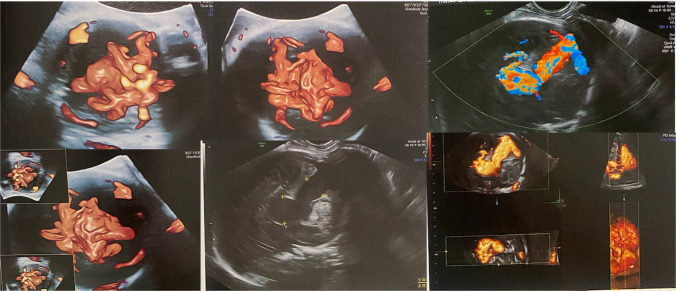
Obstetric Doppler ultrasonography at 32 + 5 weeks of gestation demonstrating a large Vein of Galen Malformation, measuring 10,500 mm^3^ in volume and a falcine sinus width of 10.5 mm
